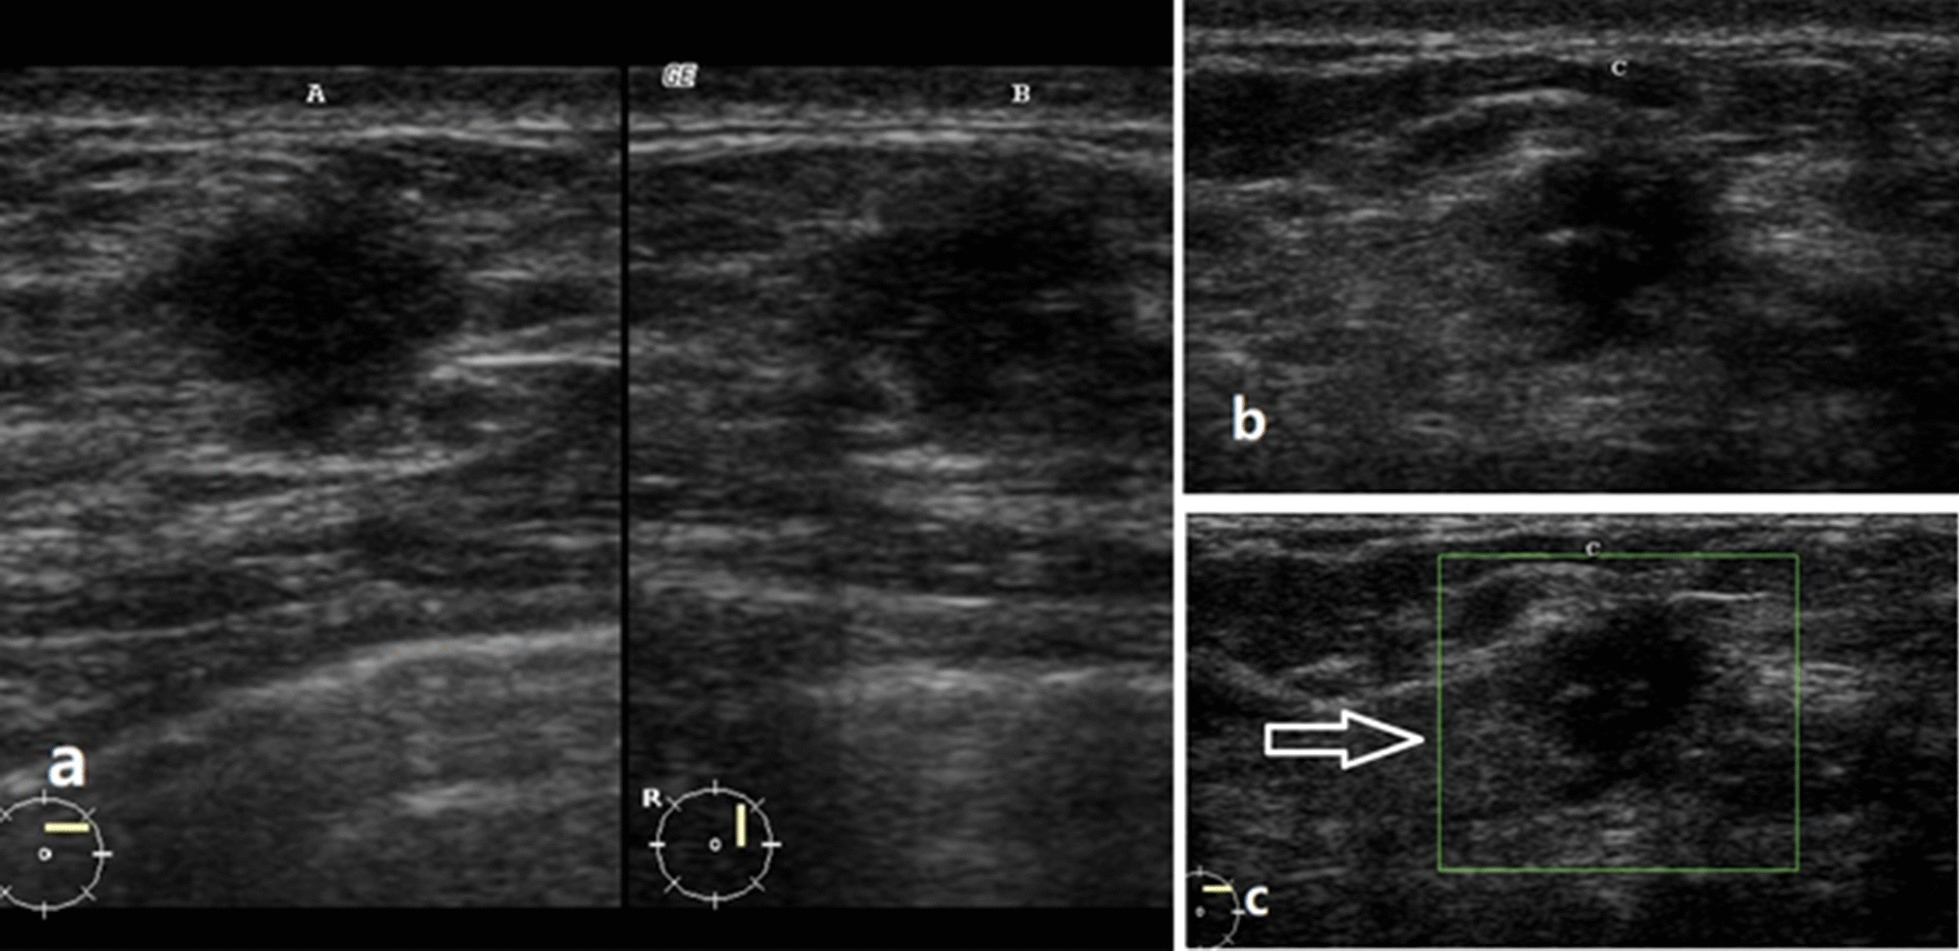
a, b transverse, sagittal and coronal US showed the lesion at 1 o 'clock in the right breast. It was a 10 × 10 × 8 mm irregular, indistinct and heterogeneous hypoechoic mass, which had no posterior shadowing. c Color Doppler shows no vascularity in the mass (white arrows)
